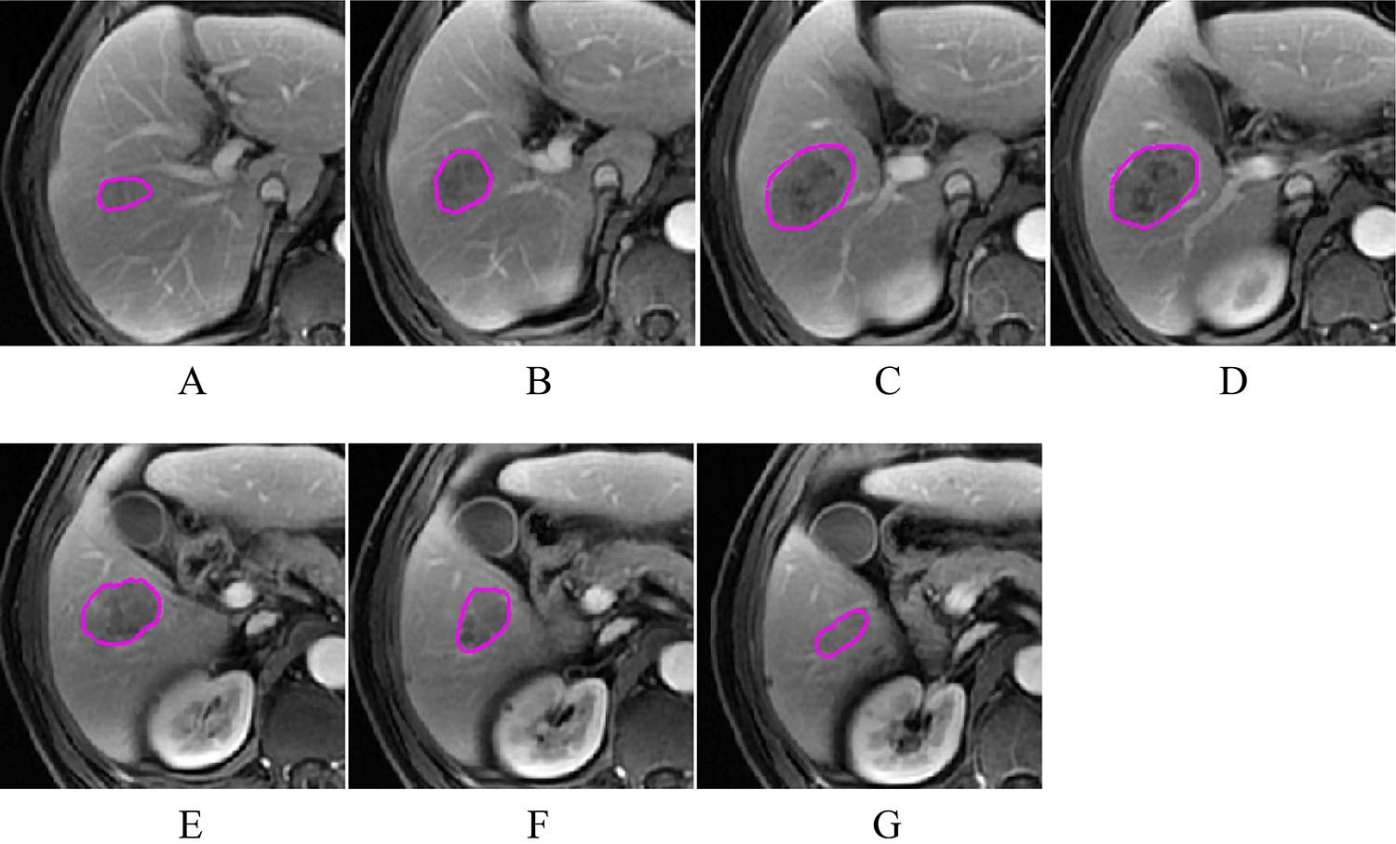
The region of interest (ROI) was delineated layer by layer (a–g) on the DCE-MRI images to obtain the volume of interest (VOI) for radiomics feature extraction.
